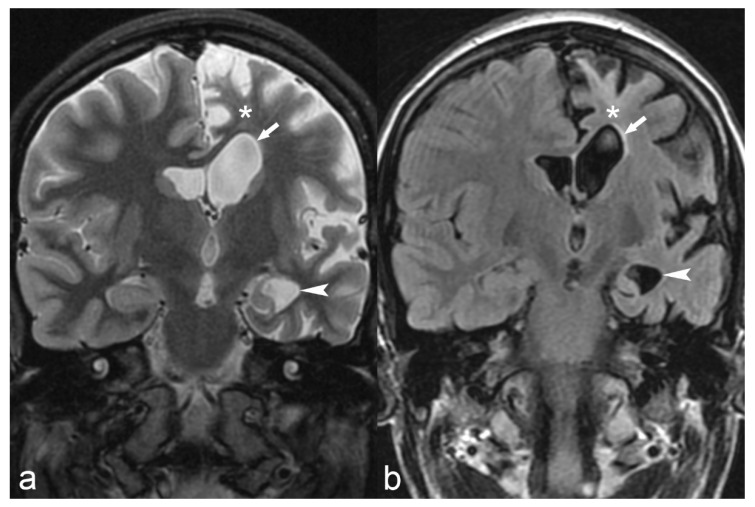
18-year-old female with medically refractory epilepsy. Coronal T2 (a) and FLAIR (b) images show hemispherical parenchymal volume loss and cortical thinning (asterisk) on the left side with resultant ex vacuo dilatation of the frontal (arrow) and temporal horns (arrowhead) of the left lateral ventricle, consistent with Rasmussen’s encephalitis.
